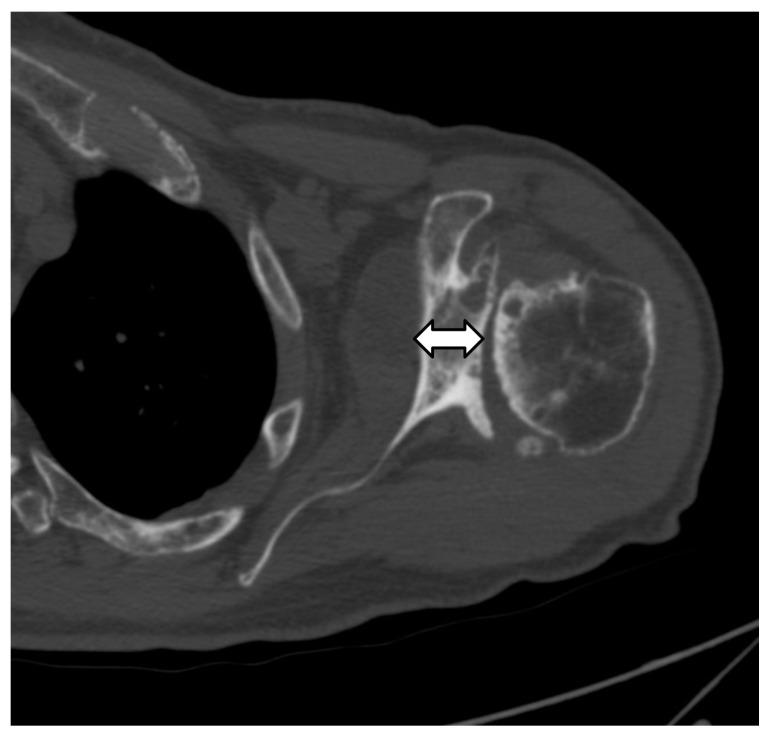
Axial CT image shows decreased glenoid bone stock (double arrow) due to glenoid erosion and retroversion.
